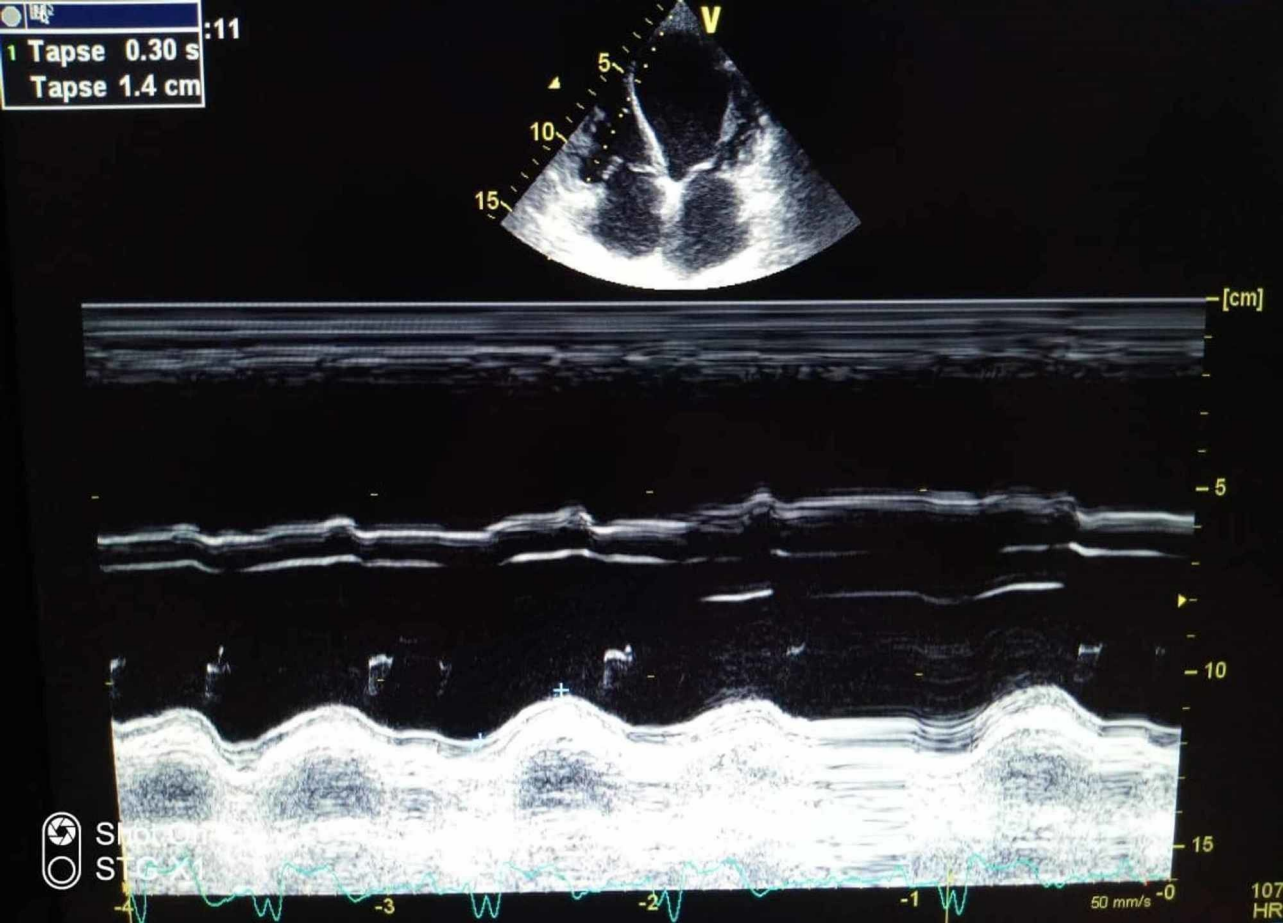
Transthoracic echocardiography showing RV systolic dysfunction RV: right ventricle
